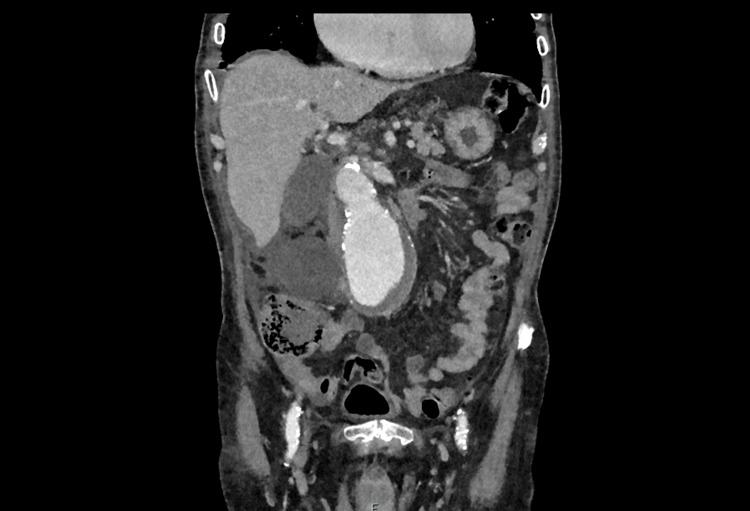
CT angiogram showing a coronal view of a large 7.5 cm infrarenal abdominal aortic aneurysm with incidental right-sided large renal cyst.
